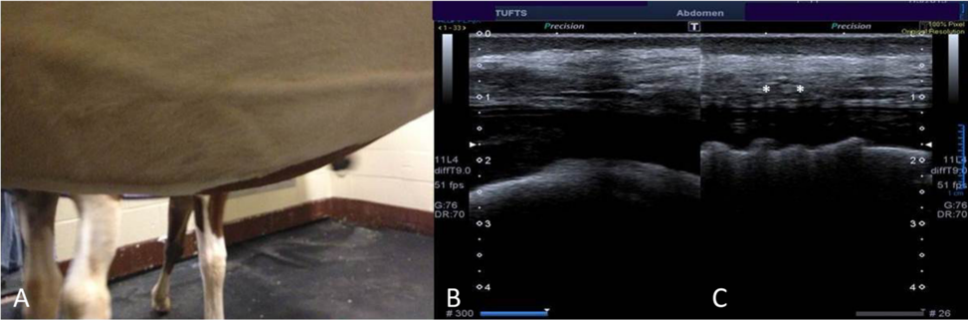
Postoperative (2 years) image of the ventral midline following mesh hernioplasty (A) with corresponding ultrasound images (B, C) documenting mature fibrous tissue formation throughout the hernioplasty site. Hypoechogenic shadow artifacts (*) representing remnants of the silk mesh are still evident. Numbers at the edge of the ultrasound images represent 1-cm depth increments.
